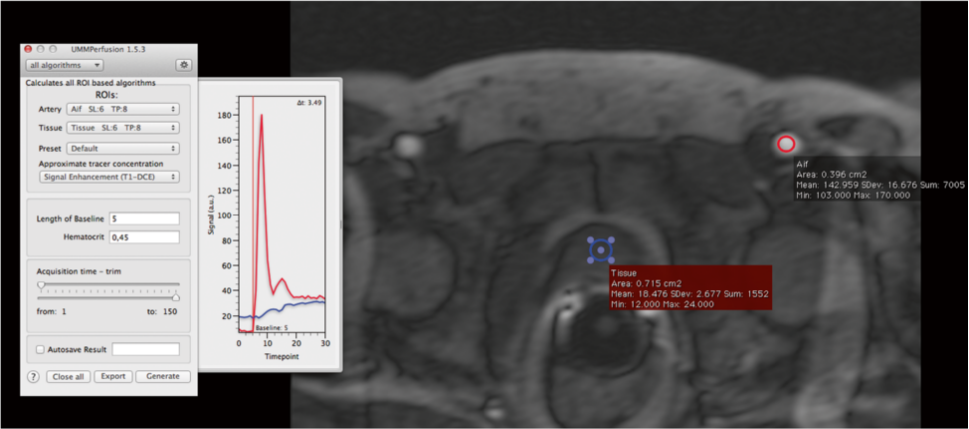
Display of the GUI and the AIF (*red curve*) for example DCE MRI data set of the prostate. Here, the AIF was selected in one of the Iliac artery. The *blue curve* is the mean signal intensity curve of prostate tissue corresponding to the blue ROI
